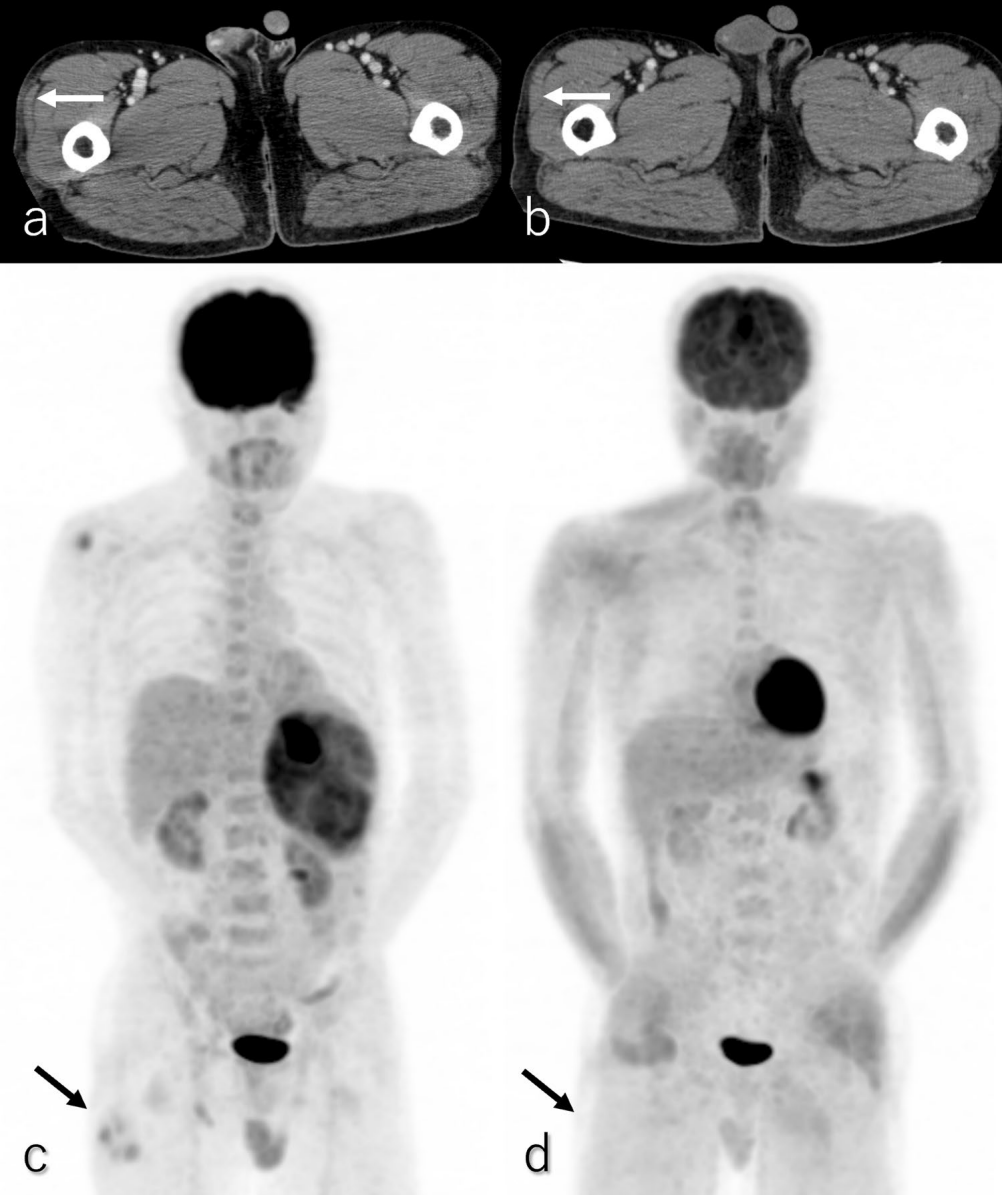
A 59-year-old male patient with acinar cell carcinoma (ACC), the same patient as in Figs. 8 and 9. a and b Dynamic contrast-enhanced CT (DCE-CT) in the pancreatic phase, c and d maximum intensity projection images of ^1^⁸F-fluoro-2-deoxy-D-glucose positron emission tomography with CT (^1^⁸F-FDG-PET/CT). Images (b) and (d) are obtained 7 months after the examinations shown in (a) and (b), respectively. The tumor is located in the pancreatic tail, measuring 15 cm in diameter, and exhibits expansile growth with well-defined margins. DCE-CT reveals an ill-defined, hazy appearance of the subcutaneous fat in the right thigh (arrow). ^1^⁸F-FDG-PET/CT demonstrates slightly increased ^1^⁸F-FDG uptake, with a maximum standardized uptake value of 2.14 in this region. These findings are consistent with pancreatic panniculitis (PP), characterized by subcutaneous fat necrosis associated with ACC. Following the surgical resection of the ACC, the ill-defined hazy appearance and ^1^⁸F-FDG uptake resolved, indicating an improvement in PP associated with the ACC
